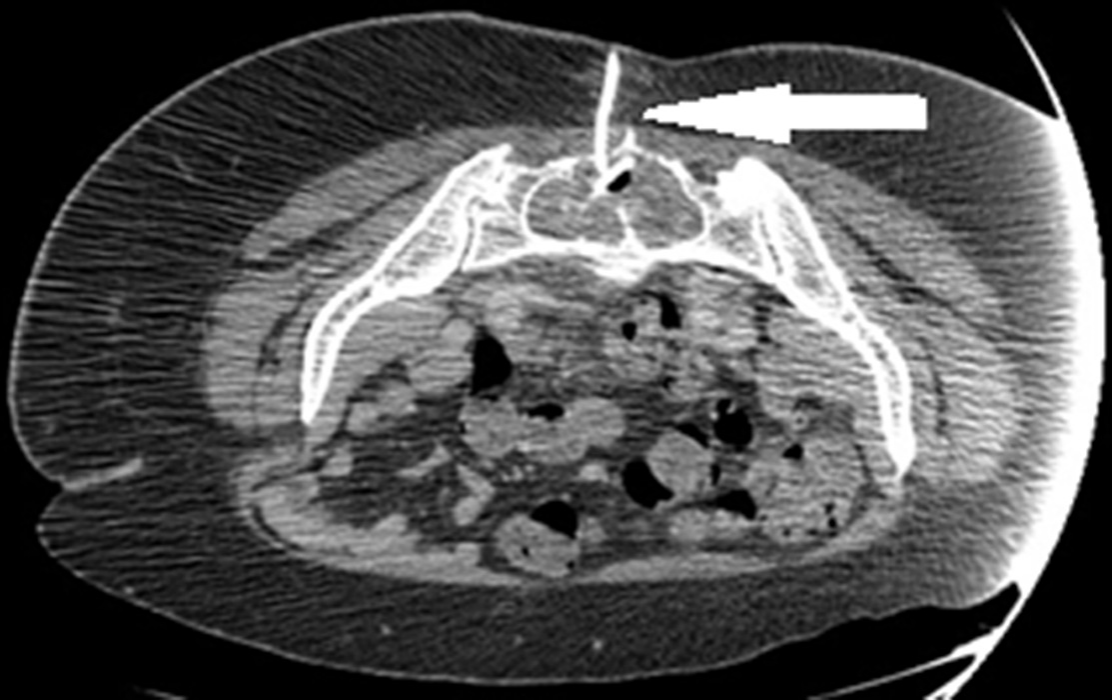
CT-guided drain insertion. The pigtail drain (arrow) has been successfully sited in the abscess within the sacral cyst, facilitated by the markedly thinned sacral lamina, permitting passage of a standard drainage access needle.
